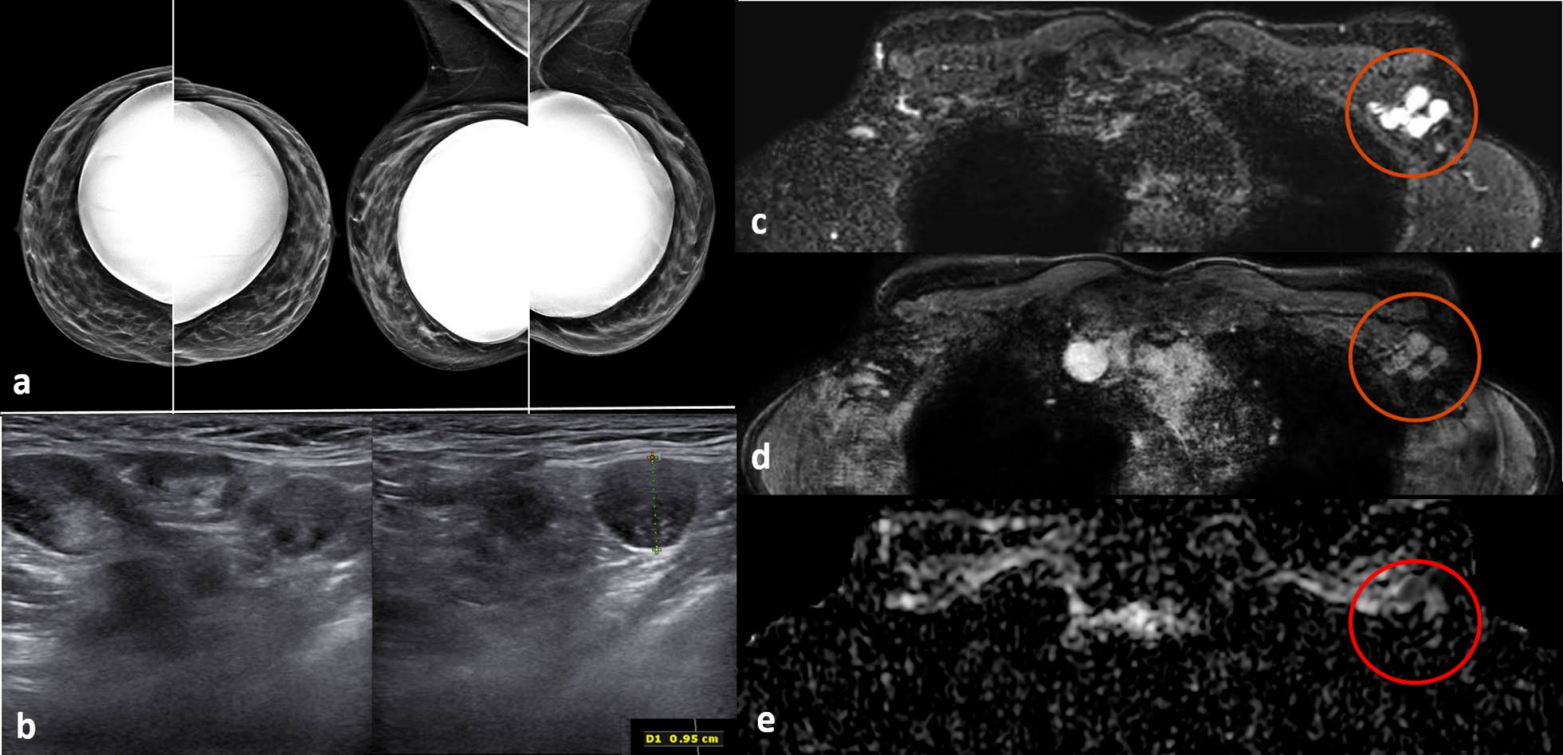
Screening mammography performed in a 44-year-old woman with a positive family history for breast cancer (mother and aunt), bearing implants for aesthetic purposes. Mammography (a) was considered negative. Breast ultrasonography was also performed because of her family history and high breast density (ACR category *d*). While ultrasonography was negative for both breasts, multiple round, enlarged, hypoechoic lymph nodes (measuring up to 1 cm in axial diameter), with a thickened (< 3 mm) cortex, were seen in the left axilla (b). There were no skin changes and there was no history of any infection or trauma. On the right side, axillary lymph nodes were normal. Because of her family history and the presence of breast implants, magnetic resonance imaging was performed (c T2-weighted short-time inversion recovery; d fat-sat contrast-enhanced T1-weighted gradient-echo; e apparent diffusion coefficient map). No suspicious mass or non-mass lesions were seen in both breasts. Implants showed no signs of rupture (not shown). In the left axilla, multiple enlarged lymph nodes were well visible in c and d (red circles); on the apparent diffusion coefficient map (e, red circle), they mainly exhibited low signal (restricted diffusivity). When an ultrasound-guided biopsy of the most suspicious lymph node was proposed, the patient mentioned that she had a Comirnaty COVID-19 vaccination one week before in the left arm. The attending radiologist was more than surprised to hear this, as at that time, a COVID-19 vaccination was only administered to people older than 70 years. Follow-up performed four weeks after the second vaccination was negative and showed no residual enlarged lymph nodes
